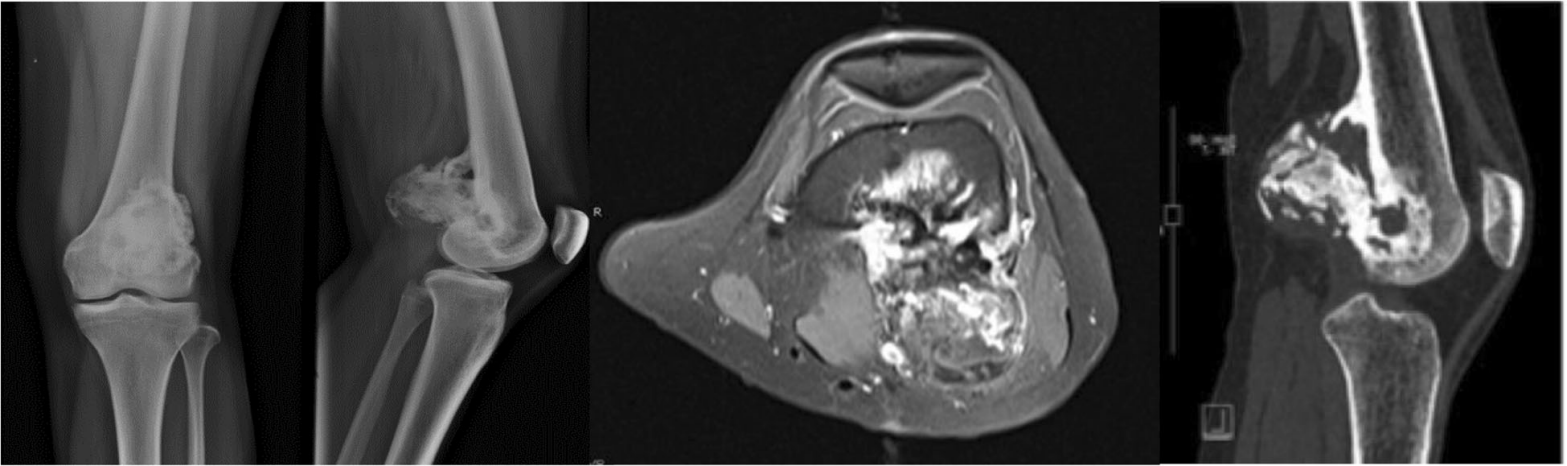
27-year-old female, parosteal osteosarcoma left distal femur (a) Plain film images of both knees demonstrating bony mass extension from the posterior part of the distal femur. b Axial MRI reveals infiltrative intramedullary lesion and soft tissue component showing heterogenous enhancement with intralesional calcification, (c) sagittal CT scan reveals bony mass involving distal femoral metaphysis from the posterior aspect
